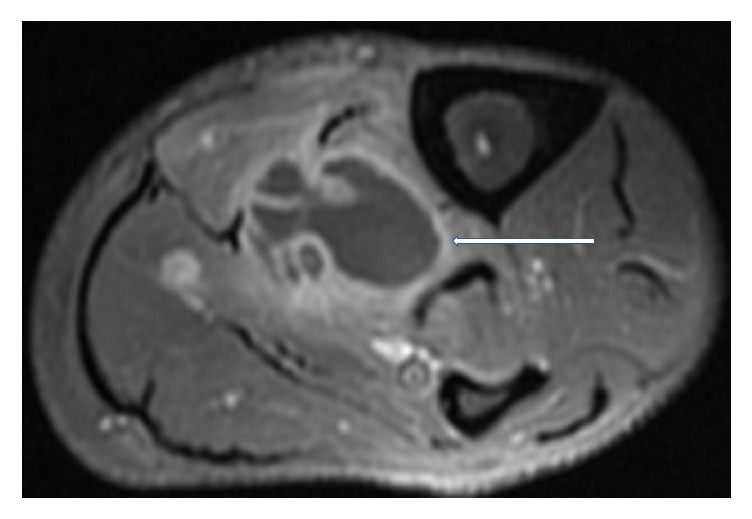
T2 weighted, fat saturated, postcontrast, axial MR image of left calf at another location, again showing a multiloculated fluid collection with associated enhancing rim and septations (white arrows) within the tibialis posterior muscle, compatible with an intramuscular abscess.
